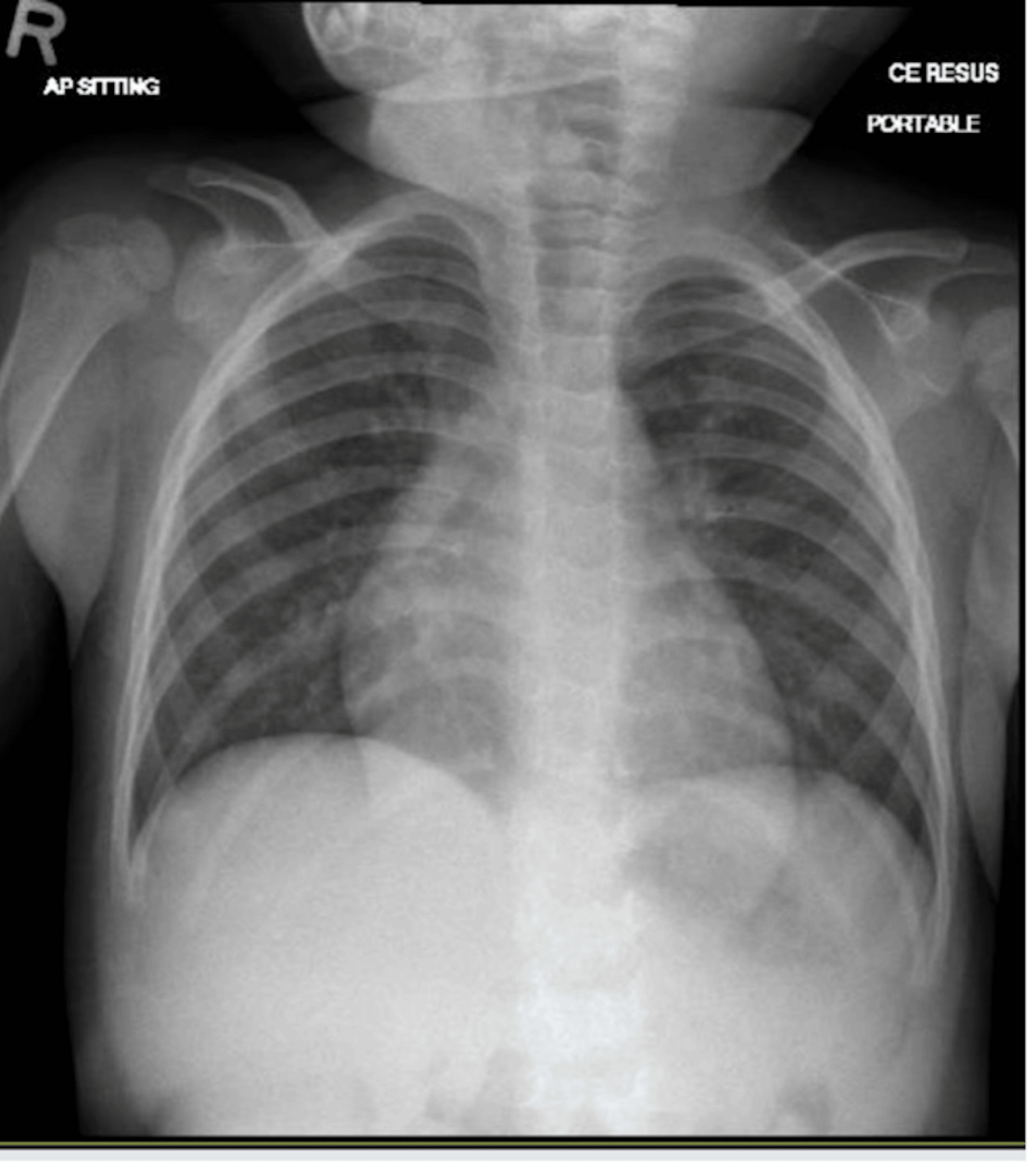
Chest radiograph Chest radiograph does not show cardiomegaly nor pulmonary oedema
